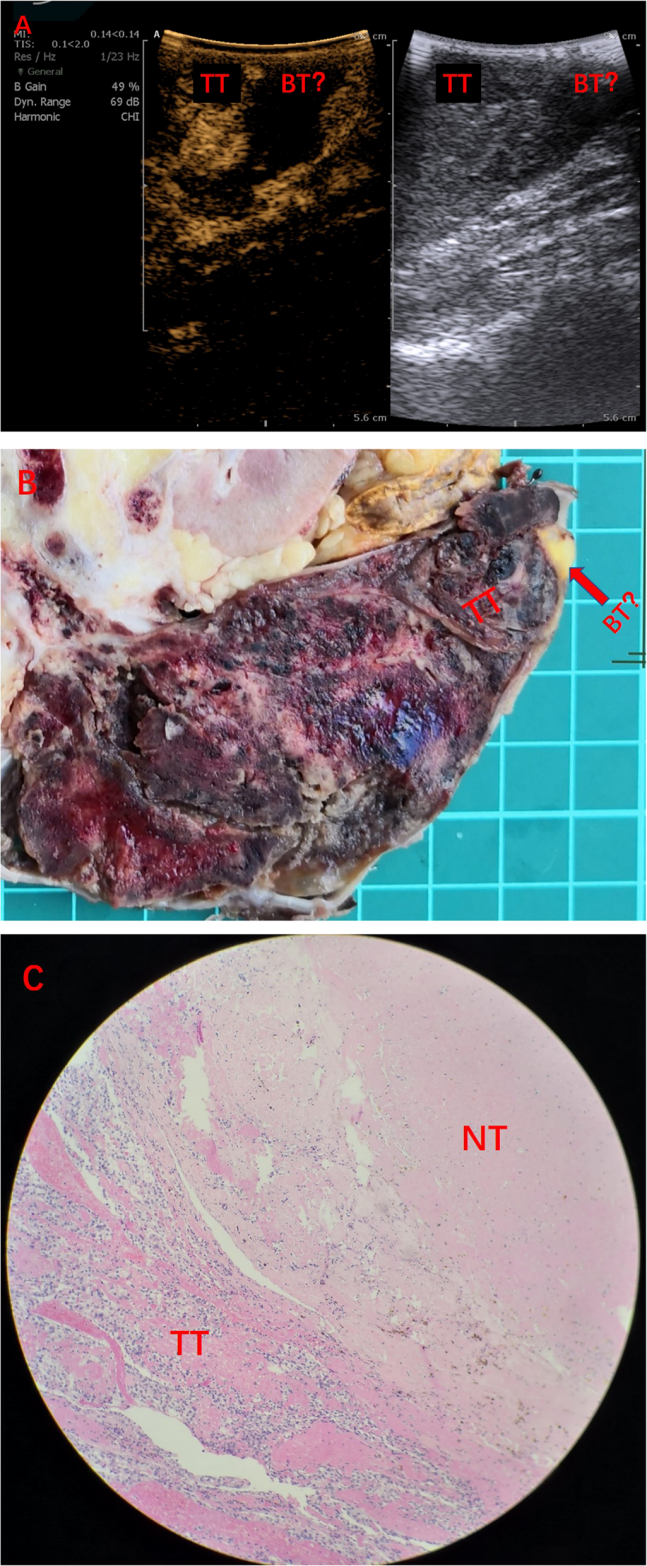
A 55-year-old female patient with a neoplasm of right kidney accompanied by IVC tumor thrombus. She underwent robot-assisted IVC transection. a Intra-operative contrast-enhanced ultrasound (CEUS) reveals that there is no perfusion area on the head side of the tumor thrombus, and co-existence of tumor thrombus and bland thrombus? b Post-operative morphology shows yellow tissue on the head side of the tumor thrombus (arrows). c Post-operative pathology reveals necrotic tissue on the head side of tumor thrombus. IVC = inferior vena cava, TT = tumor thrombus, BT = bland thrombus, NT = necrosis
